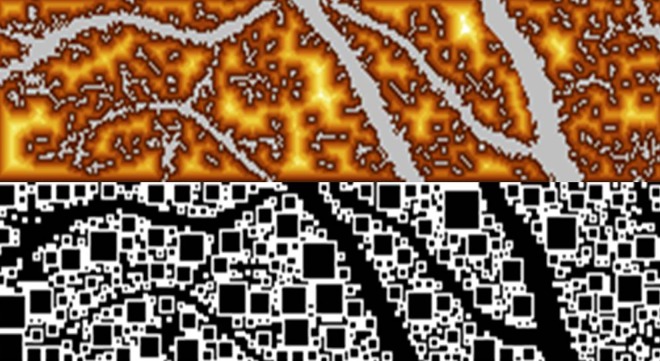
Measurement of intercapillary distance in perfusion image with scanning laser Doppler flowmetry and calculation of intercapillary distance using automatic full field perfusion image analyser
